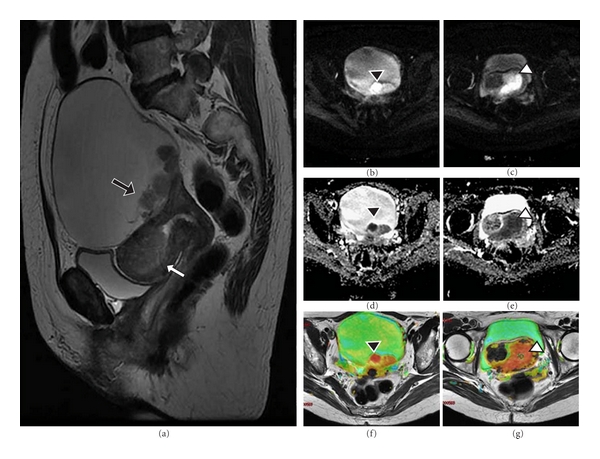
Synchronic ovarian serous cystadenocarcinoma and endometrium adenocarcinoma (stage IC) in a 60-year-old woman. (a) *Sagittal T2-WI image* shows a unilocular cystic mass with solid mural nodules (black arrow) of heterogeneous hyperintensity. There is an intermediate signal intensity mass filling the endometrial cavity (white arrow). ((b) and (c)) *DWI*: show high signal intensity corresponding to mural nodules (black arrowhead) and an ill-defined slightly hyperintense mass in the endometrial area (white arrowhead). ((d) and (e)) *ADC maps*: depict low signal intensity in the solid mural nodules (black arrowhead), with an ADC value of 0.81 × 10^−3^ mm^2^/s, while the cystic component presents values of 1.3 × 10^−3^ mm^2^/s. ADC value within the endometrial mass 0.79 × 10^−3^ mm^2^/s (white arrowhead). ((f) and (g)): Postprocessed axial images, in which fusion between DWI and T2-WI is obtained. Low ADC areas are represented in red.
